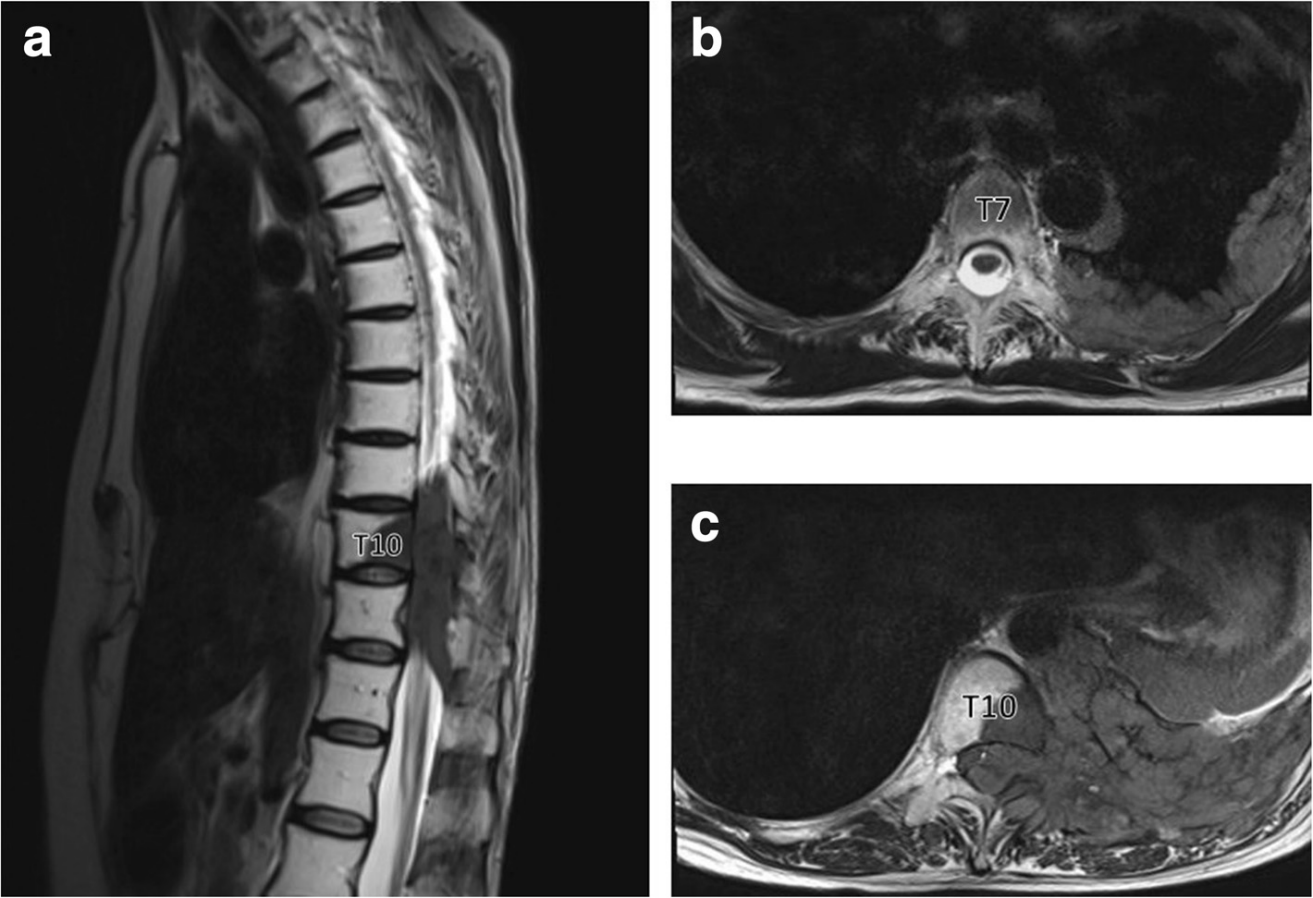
Preoperative T2-weighted magnetic resonance images of thoracic spine. a. sagittal image revealed a paravertebral mass between T9 and T12 level. b. coronal image of T7 vertebrae showed pleural mass. c. coronal image of T10 vertebrae showed spinal compression
